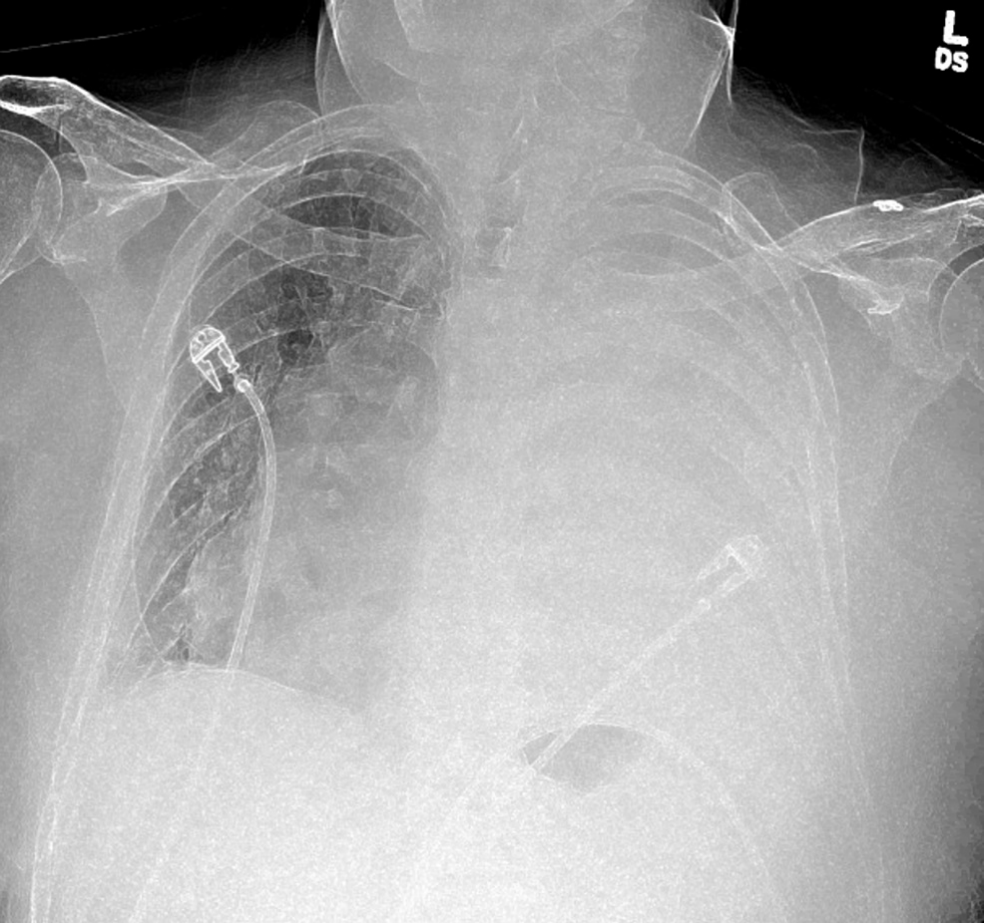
Initial chest x-ray of the patient
